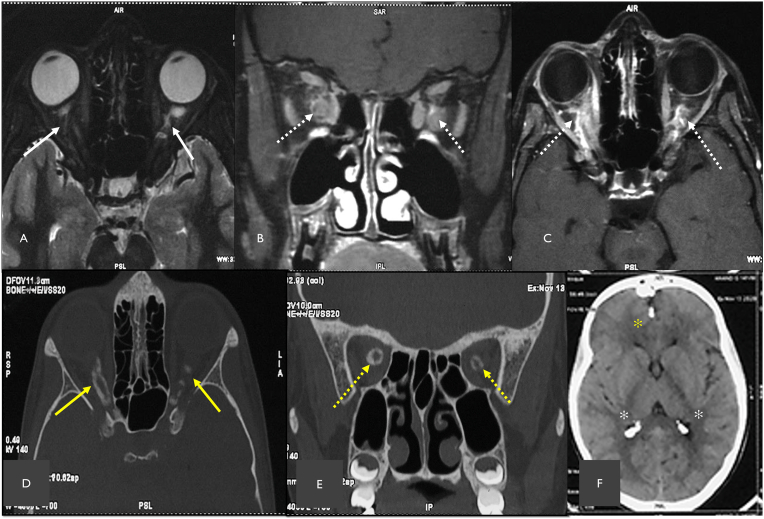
Magnetic resonance imaging of the orbit (A) T2-weighted mid-axial section shows an ill-defined lesion in the intra orbital segment of optic nerve on either side (solid white arrow); T1-weighted with gadolinium contrast of the mid orbit coronal section(B) and mid-axial section(C)shows thick and heterogenous enhancement of the optic nerve on both sides (dashed white arrows). (D) Computed tomography in the bone window of mid-axial section of orbits shows hyper-density along the optic nerve sheath of right and left optic nerve (yellow arrow); (E) shows ring shaped hyper-density encasing the right and left optic nerve (dashed yellow arrow), (F) Computed tomography of brain shows intracranial calcification of flax cerebri (yellow asterix) and choroid plexus of lateral ventricle (white asterix). (For interpretation of the references to color in this figure legend, the reader is referred to the Web version of this article.)
